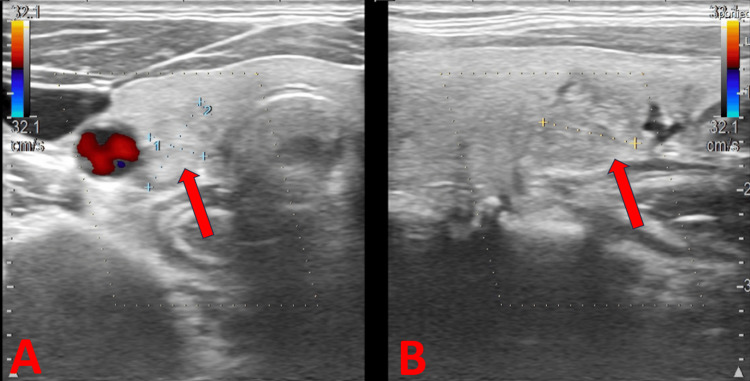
UDP (A) Focal change in the thyroid gland, transverse section. (B) Focal change in the thyroid gland, longitudinal section UDP: Doppler ultrasound
